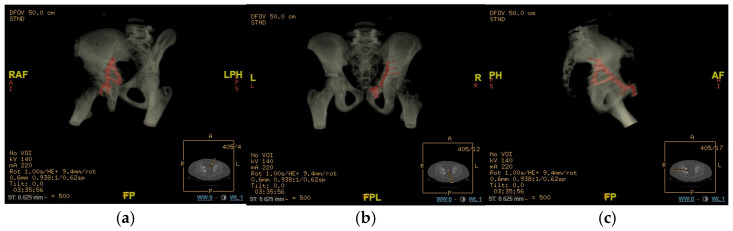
CT render of the plating area of the right acetabulum at 1 month follow-up. (a) Oblique view; (b) anteroposterior view; (c) lateral view. R = right; L = left; A = anterior; P = posterior; H = Head; F = Foot.
